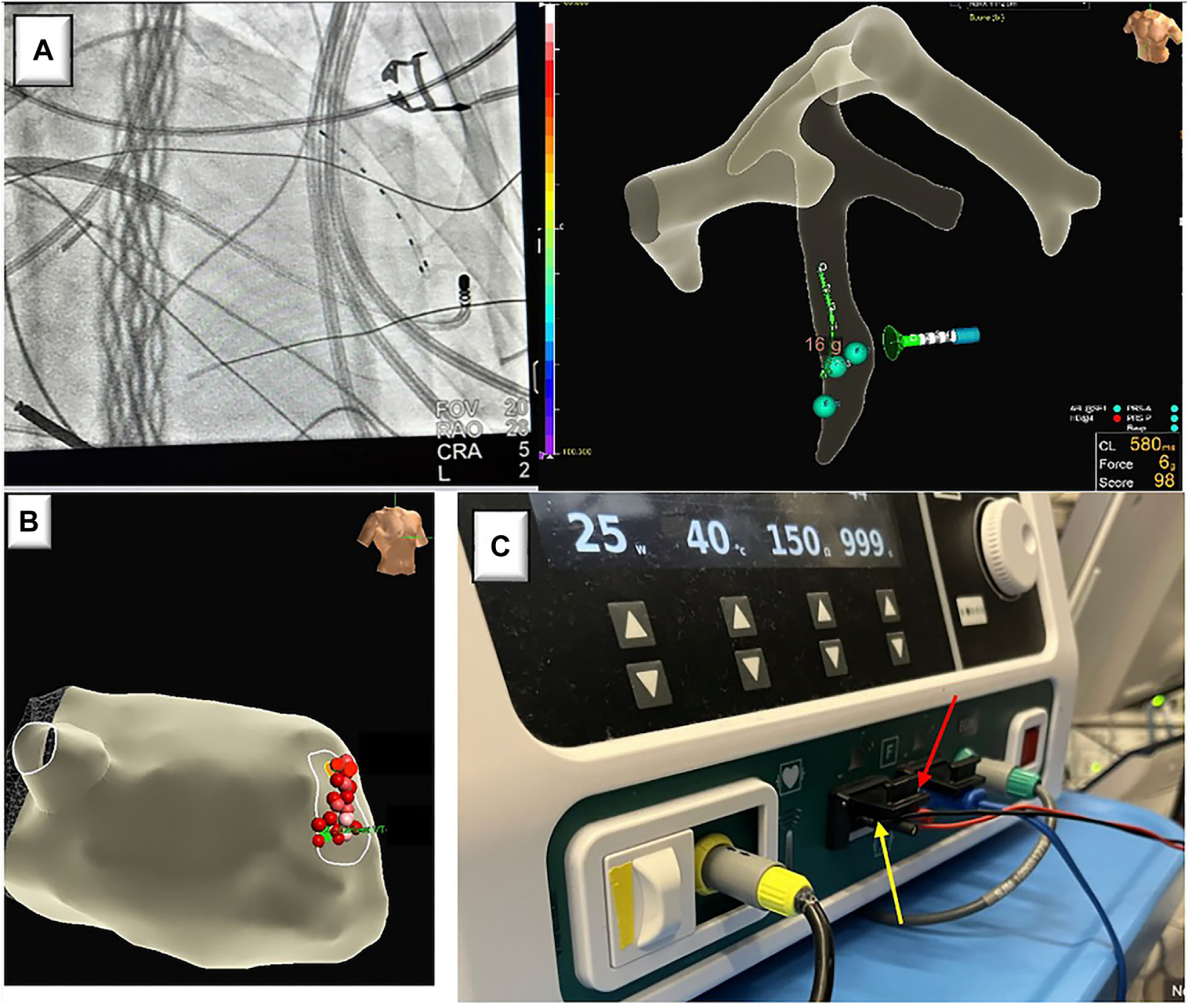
A: Radiographic and mapping system depiction of ablation vector between left ventricle (LV) endocardial ablation catheter and anterior interventricular vein–positioned multipolar catheter. B: Final lesion set created by adjusting the LV endocardial ablation catheter position in relation to the multipolar catheter. C: Generator setup for multipolar ablation with EPstar 6 (Baylis Medical) connected to the ampere return via the red jumper cable (*red arrow*) and EPstar 8 (Baylis Medical) connected to the ampere return via the black jumper cable (*yellow arrow*).
